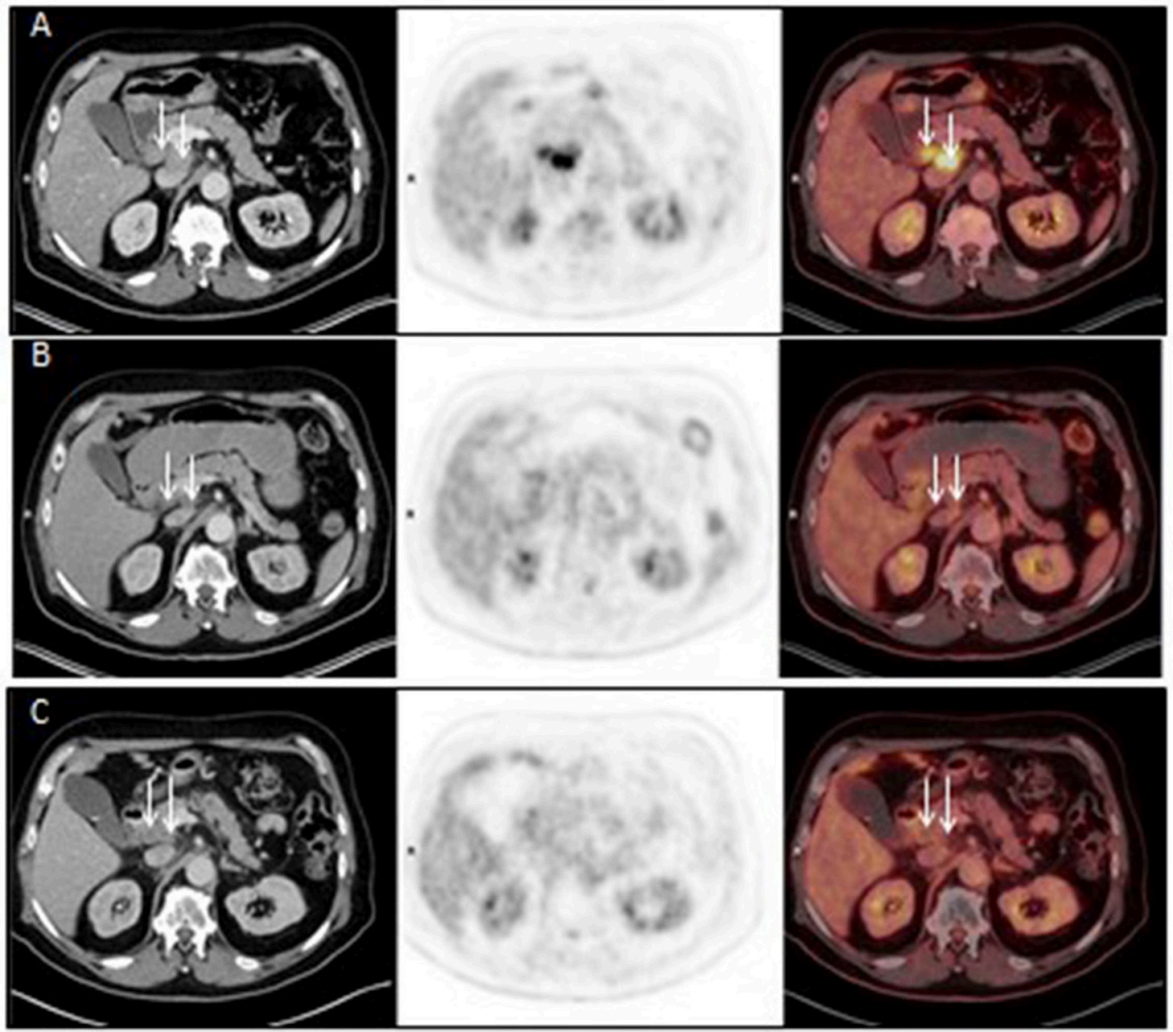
Axial CT (left panel), FDG PET (middle panel) and fused FDG PET/CT (right panel) images before Promitil with radiotherapy (A, 07Apr2016), 3 months after Promitil with radiotherapy (B, 04July2016), and on a recent re-evaluation 22 months later (C, 19Feb2018). Initial pre-therapy intense pathological uptake in two adjacent porto-caval lymph nodes (arrows) resolved after therapy, with significant interval reduction in lymph nodes dimensions.
